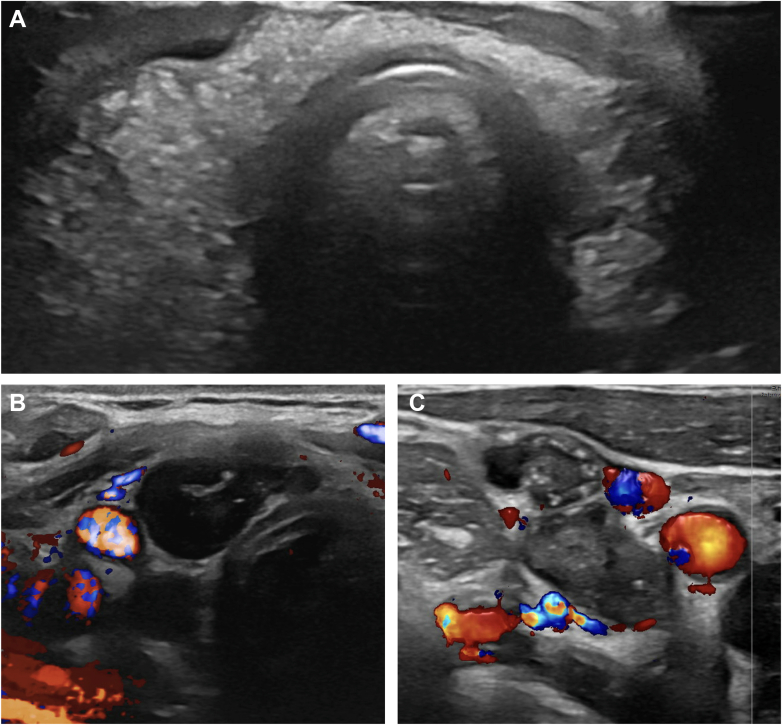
Neck ultrasound showing (*A*) heterogeneous thyroid with punctate echogenic foci. *Right* lobe measures 6.1 × 2.7 × 2.2 cm and *left* lobe measures 5.0 × 1.8 × 1.7 cm. There are also multiple hypoechoic, round lymph nodes measuring up to 1.7 cm in the longest dimension in *right* (*B*) central neck (level VI) and (*C*) lateral neck (level III) with punctate echogenic foci and absent central vascularity.
